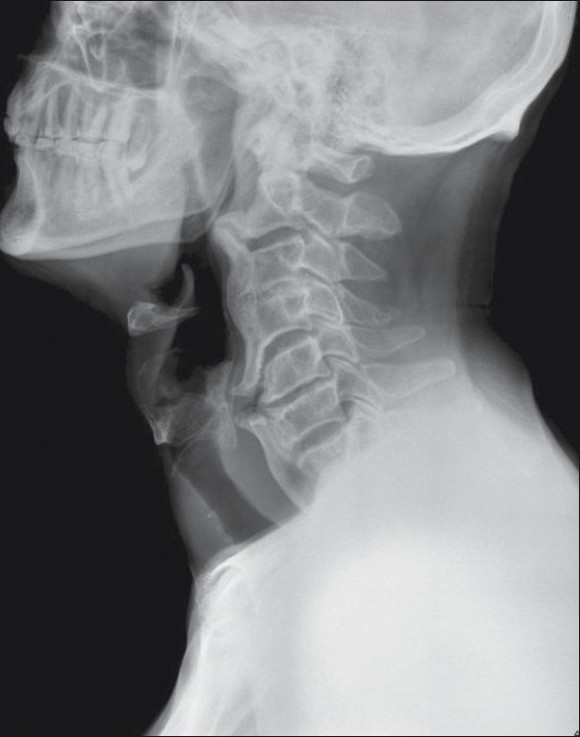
The lateral cervical spine radiograph of this young patient with dysphagia shows flowing ossification along the anterior aspect of the cervical vertebrae, with relative preservation of the disc spaces
